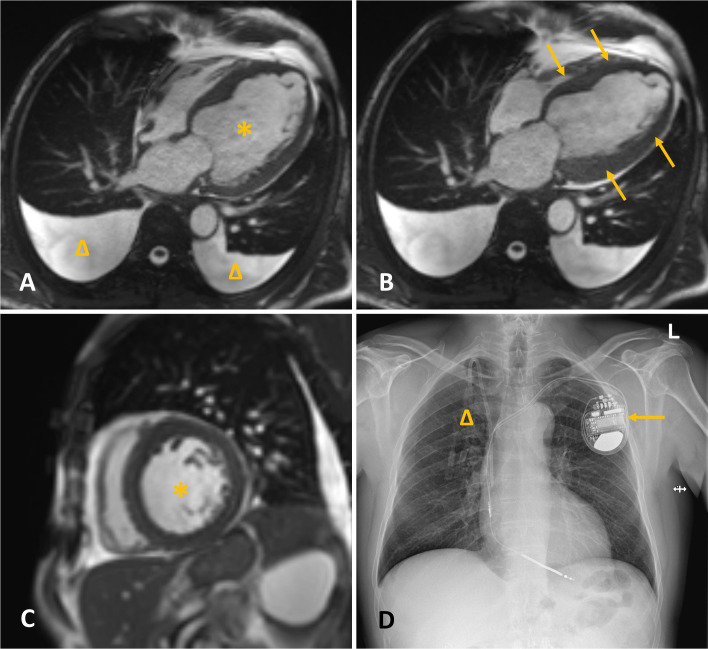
(A) Cardiac magnetic resonance imaging (CMR), steady-state free precession (SSFP) cine sequence in end-diastole (ED), 4-chamber view. This image shows an enlarged left ventricle (*) (left ventricular end-diastolic diameter 73 mm, left ventricular end-diastolic volume/body surface area 197 mL/m^2^ [reference values 47-92 mL/m^2]^) and bilateral pleural effusion (Δ). (B) CMR, SSFP cine sequence in end-systole (ES), 4-chamber view. In comparison with (B), the entire left ventricular myocardium shows severe global hypokinesia (arrows), which results in severely reduced ejection fraction (volumetric analysis showed left ventricular ejection fraction of 16%). (C) CMR, SSFP cine sequence in ED, short-axis view. In this image, a dilatation of the left ventricular cavity is seen (*), compared with the right ventricle. (D) Chest x-ray in an erect position, postero-anterior projection. A two-chamber cardiac resynchronization therapy device (arrow) and dialysis catheter (Δ) are shown, both in an adequate position. The cardiovascular silhouette is enlarged, lung fields show normal transparency and no pleural effusion.
